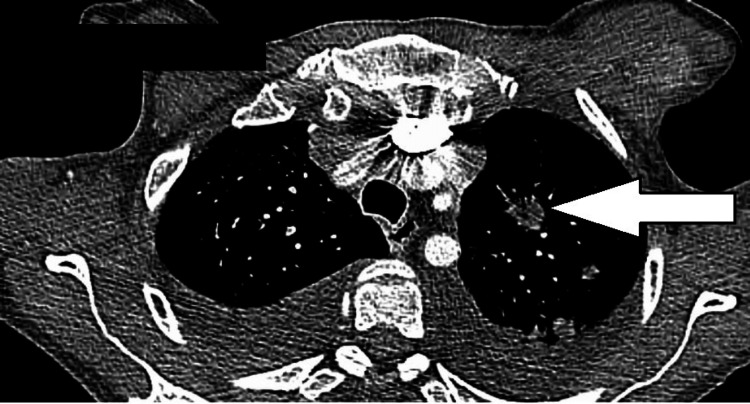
Left-sided pulmonary nodule noted on CT chest.
